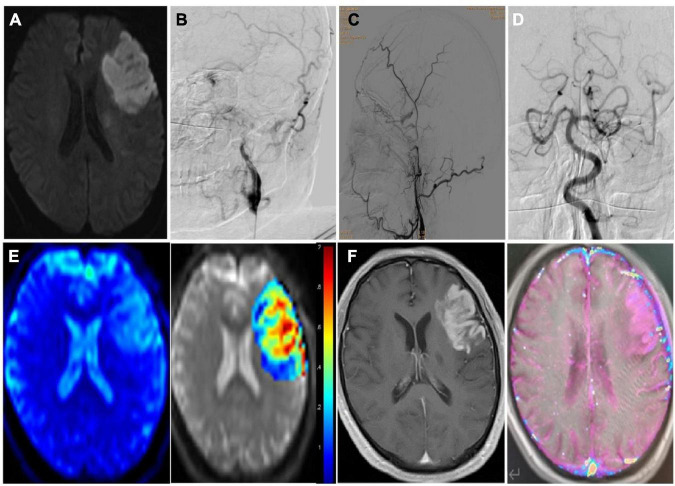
Imaging data of a patient with subacute ischemic stroke with hemorrhagic transformation after endovascular therapy. (A) Seven days after stroke onset, diffusion weighted imaging sequence of MRI shows subacute cerebral infarction in left frontal temporal lobe. (B–D) Cerebral angiography revealed long-segment occlusion of the left internal carotid artery, but the left middle cerebral artery and its branches remain patent. (E) The pseudo color image (left) and *post*-processing image (right) of the ASL (arterial spin labeling) sequence are shown with the cerebral blood flow of 1.9. (F) The original image (left) and the *post*-processing image (right) of the DCE (dynamic contrast enhanced) MRI sequence are shown with the Ktrans of 0.16/min).
